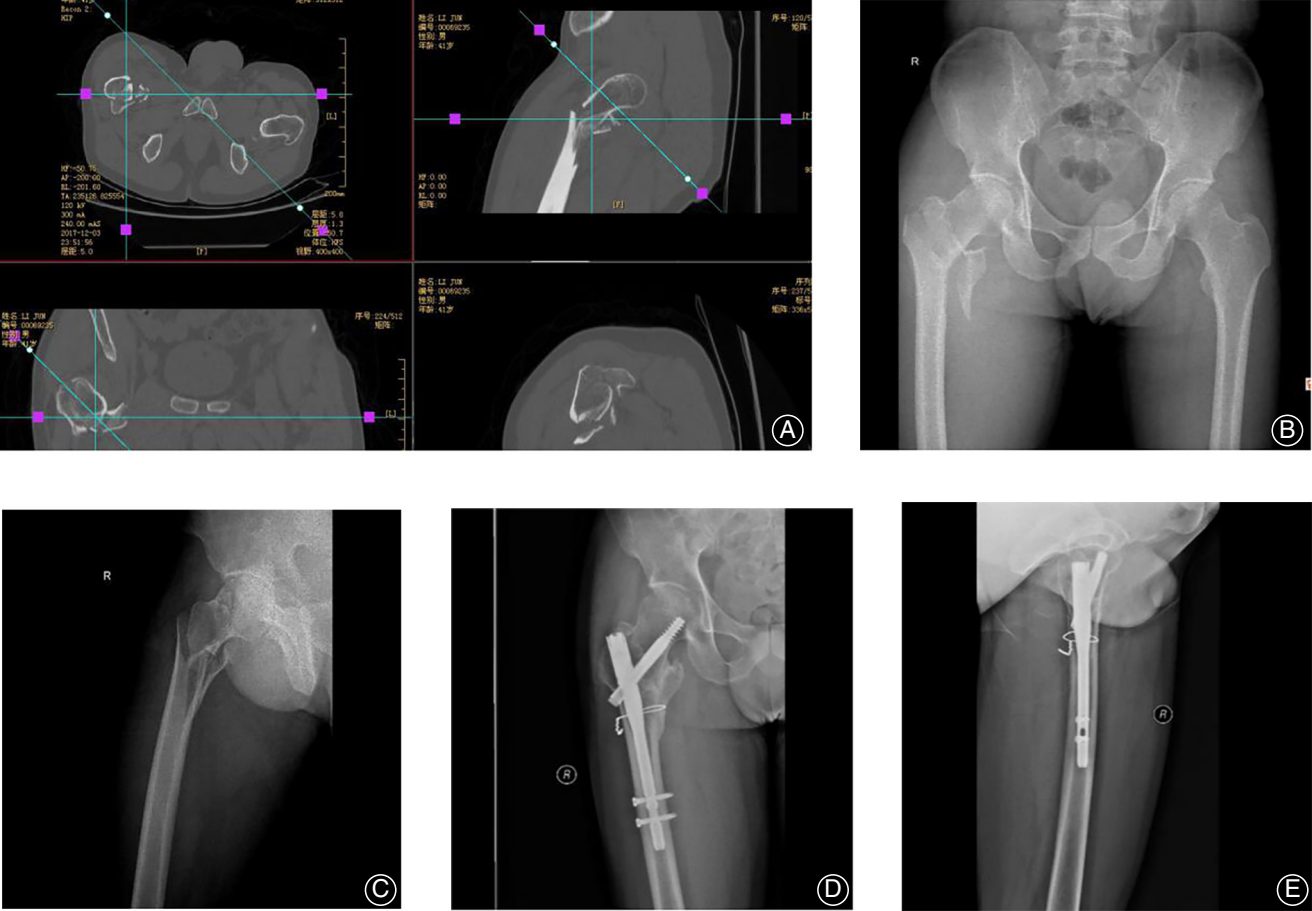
A 41‐year‐old male patient with trochanteric fracture was treated with intramedullary nail fixation and steel wire encircling fixation. (A): preoperative CT and reconstruction showed obvious fracture displacement, (B, C): preoperative X‐ray positive and lateral position showed serious lateral wall injury. (D, E): After operation, X‐ray positive and lateral position showed anatomical reduction and good fixation of fracture.
